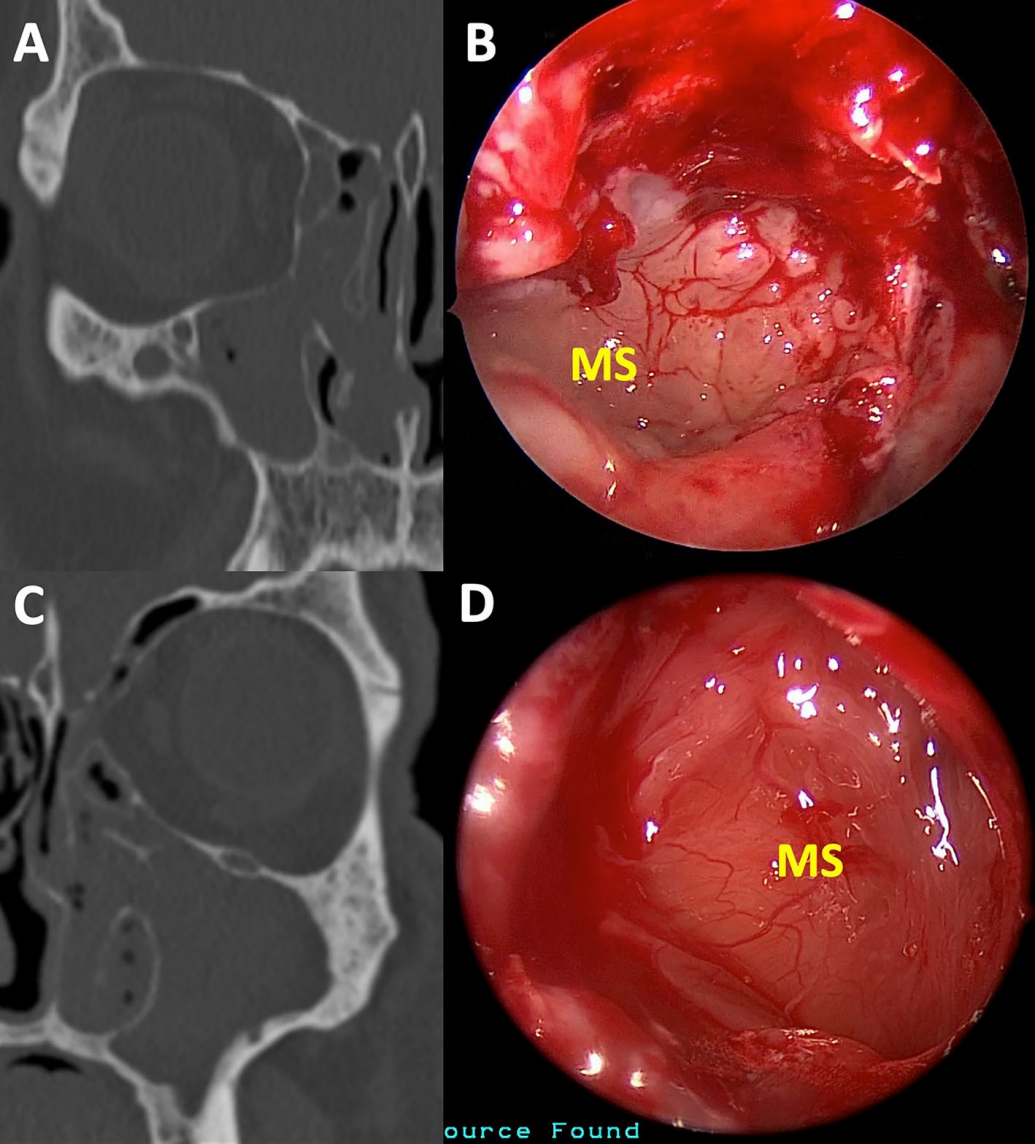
Examples of unilateral non‐odontogenic rhinosinusitis cases without maxillary sinus (MS) papillary edema. (A) Computed tomography (CT) showing right‐sided near‐complete opacification of the maxillary and anterior ethmoid sinuses in the setting of chronic rhinosinusitis with nasal polyps. (B) 70° nasal endoscopic view of the right MS lumen after maxillary antrostomy in the patient from (A). While edema was present, there were no papillary changes to the mucosal edema. (C) CT showing left‐sided maxillary and ethmoid sinus opacification in the setting of purulent non‐odontogenic rhinosinusitis without nasal polyps. (D) 70° nasal endoscopic view of the left MS lumen after modified endoscopic medial maxillectomy in the patient from (C). While the sinus walls were edematous, no papillary surface changes were seen.
